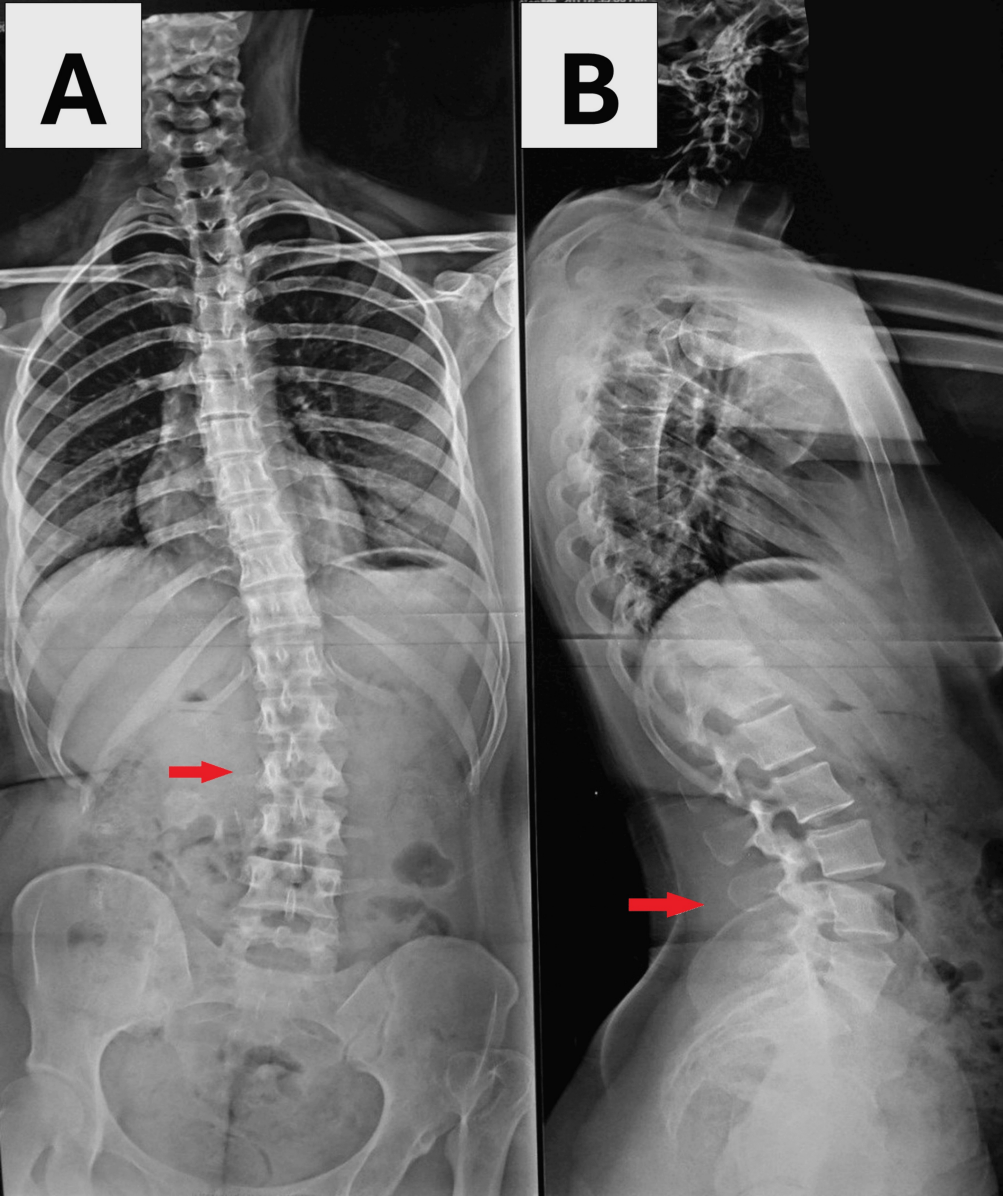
Anteroposterior and lateral radiographs of a 42-year-old female patient in our study A, B: left congenital hip dislocation, suffering from severe scoliosis (red arrows)
